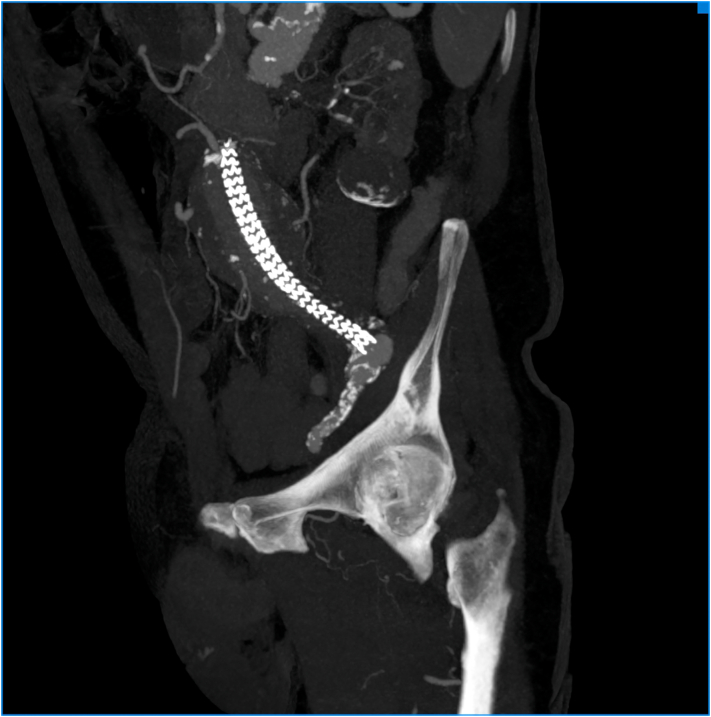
Preoperative CT Angiography showing left iliac aneurysm.
